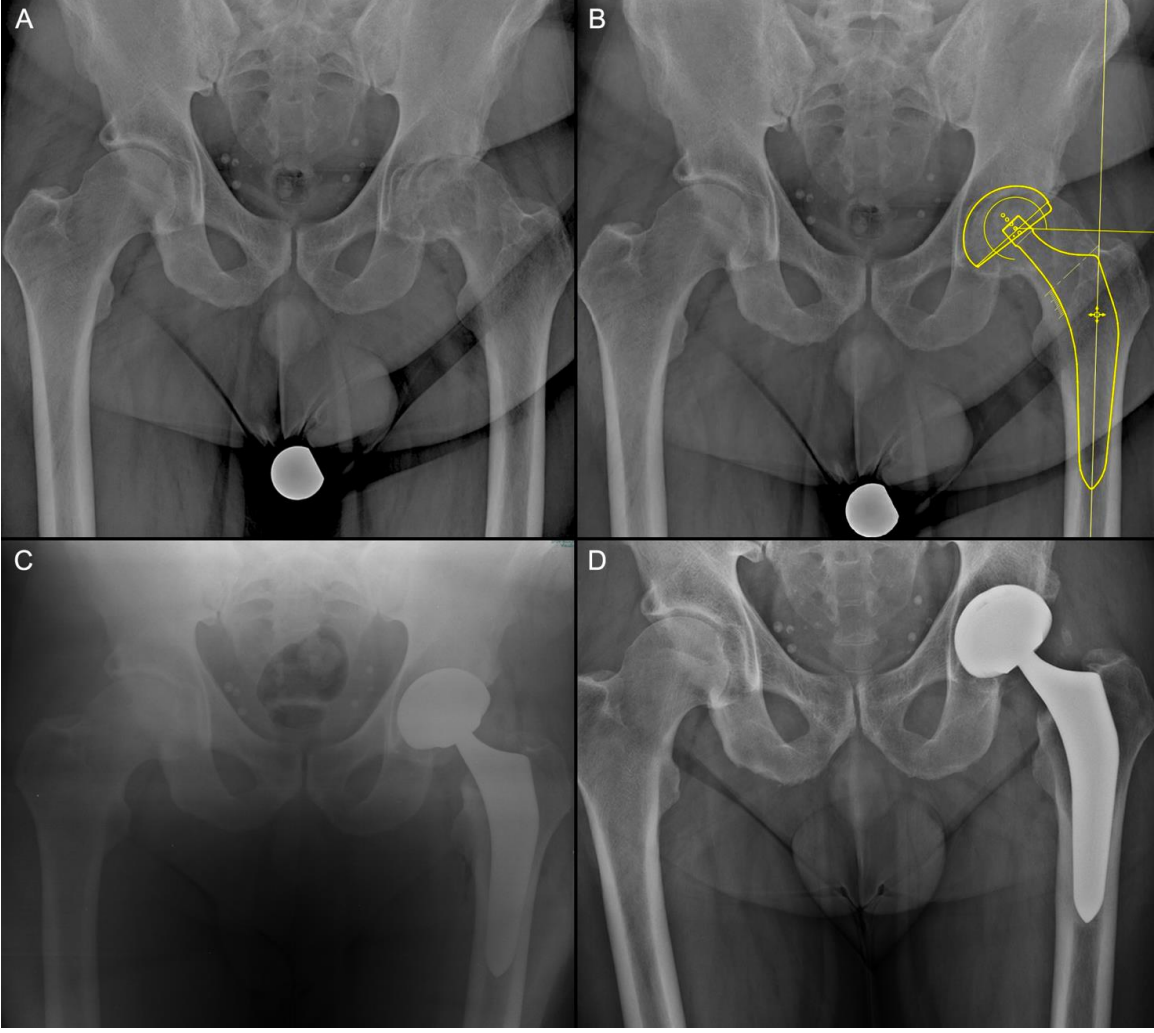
Male, 67y.o. (A) Preoperative Radiograph of the hip and pelvis. (B) Digital Templating; (C) Post-Operative Radiographic control. (D) 7 years follow-up radiograph.
